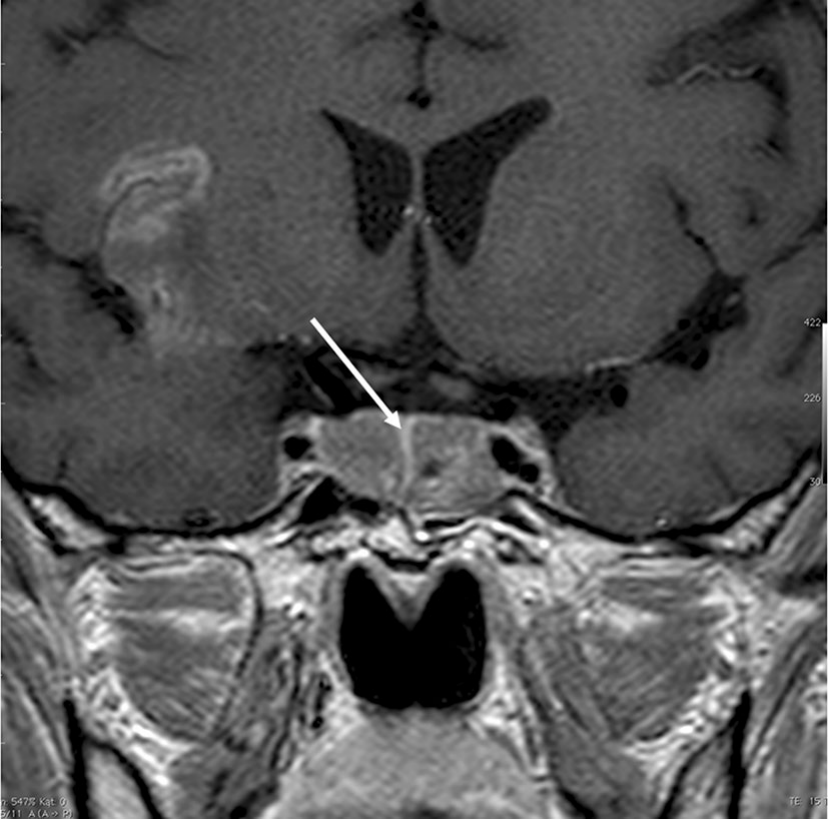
Case no. 13, T1 weighted image after GD-DTPA. Separate tumors were identified in the MR imaging and intraoperatively, the arrow indicated flattened pituitary gland
